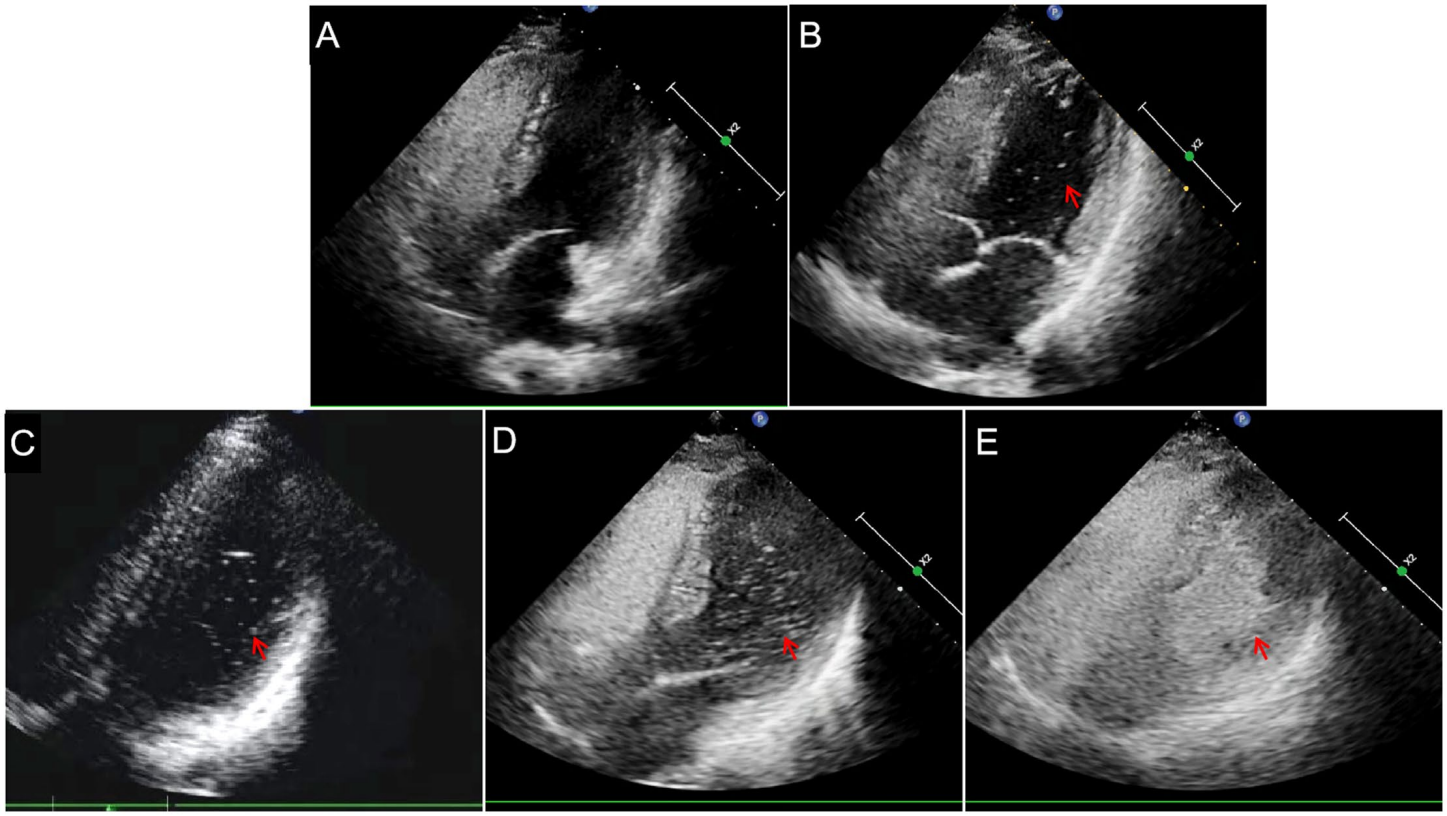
Semi-quantitative grading of right-to-left shunts in contrast transthoracic echocardiography. (A) Grade 0_TTE_ = negative. (B) Grade I_TTE_ = 1–10 microbubbles (arrow). (C) Grade II_TTE_ = 11–30 microbubbles (arrow). (D) Grade III_TTE_ = >30 microbubbles (arrow) or (E) the left atrium is nearly filled with microbubbles (arrow).
